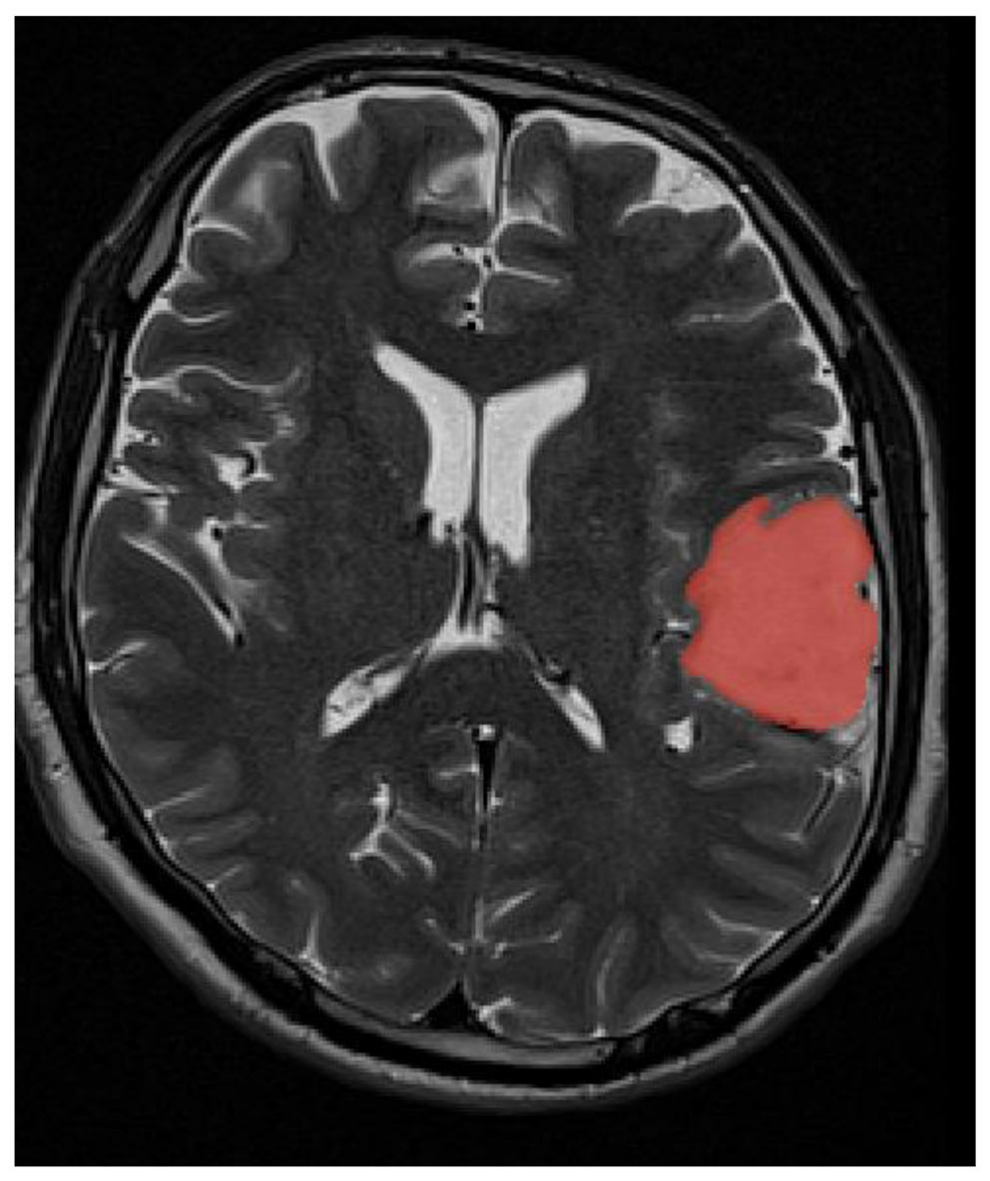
LGG after volumetric segmentation in T2 weighted MRI.
